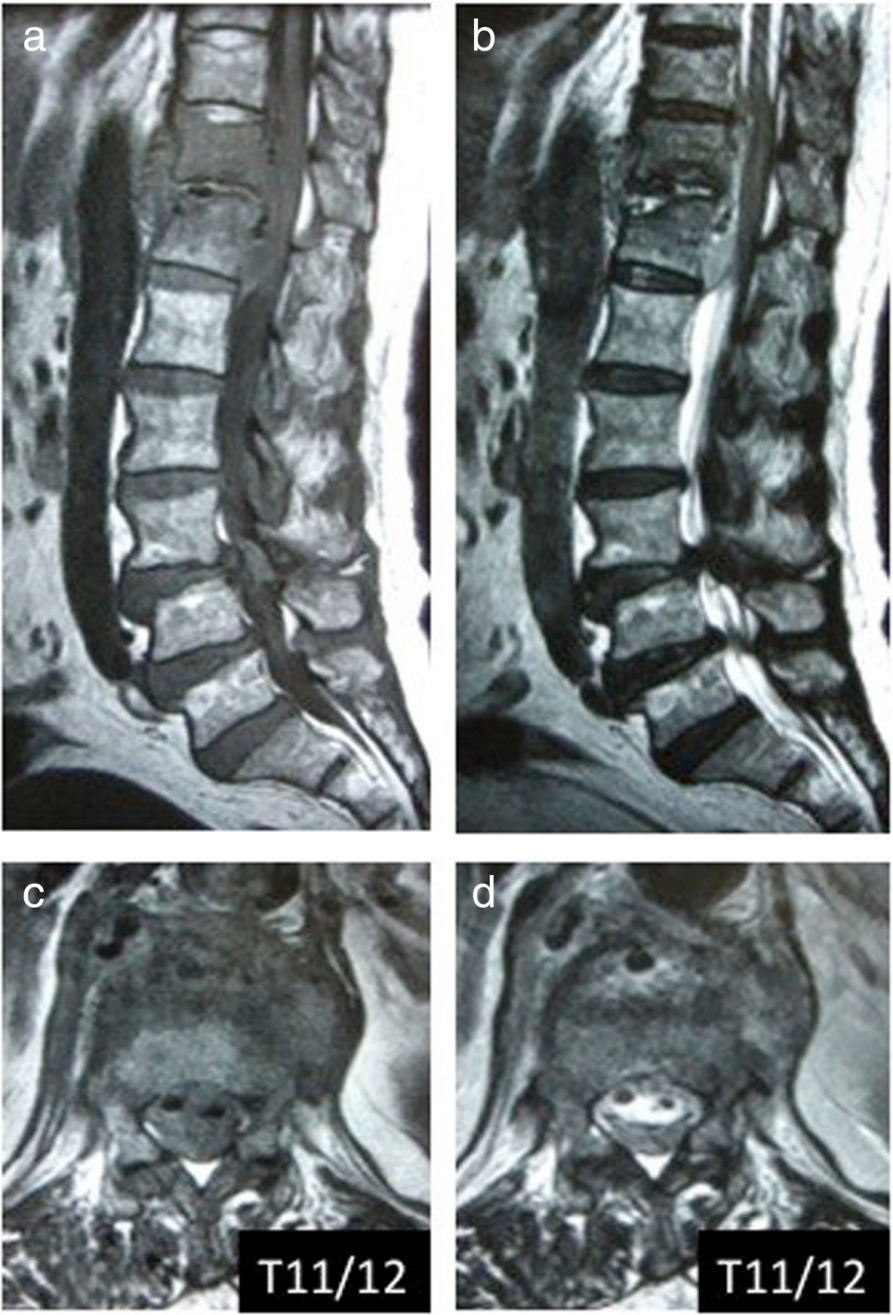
Magnetic resonance imaging sagittal and axial images on initial admission. Involvement of the T11/12 disc space and adjacent vertebral body with decreased signal intensity on the T1-weighted images (a and c) and increased signal intensity on the T2-weighted images (b and d) with a gas-containing epidural abscess are seen.
